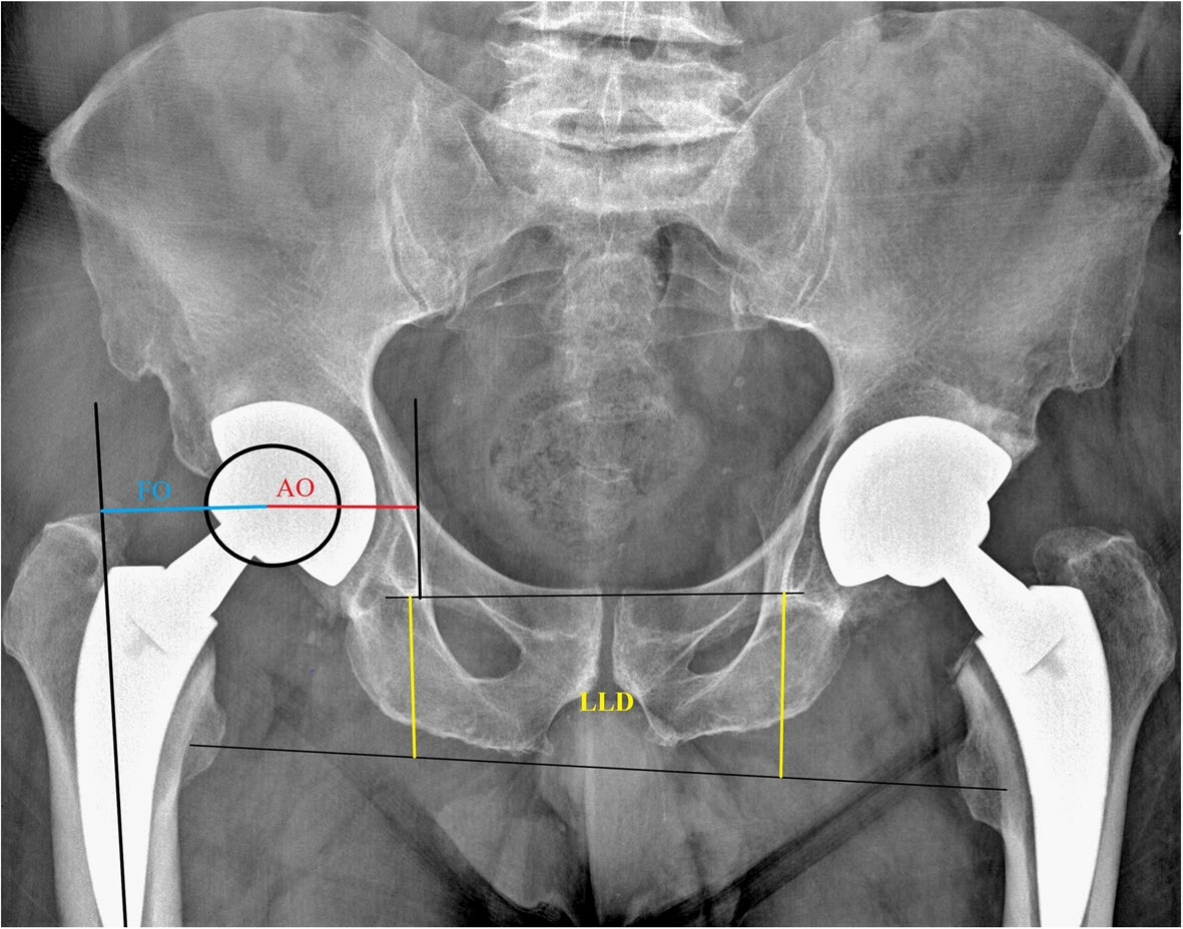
LLD, FO, and AO measurements. An example case of THA showing measurements of LLD (yellow lines), FO (blue line), and AO (red line) in posteroanterior X-ray. See text for the description of these measurements
